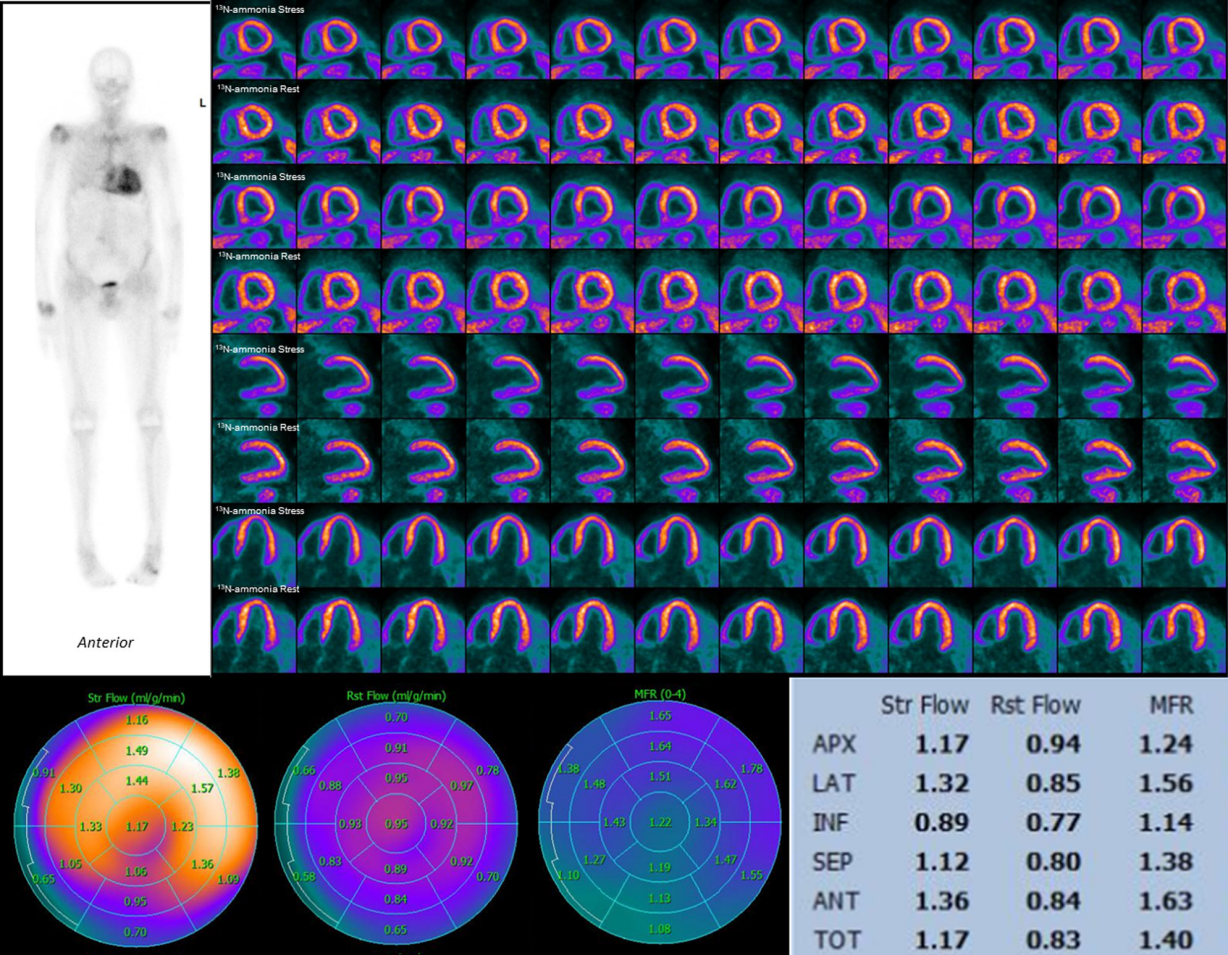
ATTR Amyloidosis and CMD. A 82-year-old patient with left ventricular hypertrophy on TTE was assessed for presence of cardiac amyloidosis. Upper left panel depicts planar whole-body scintigraphy with a bone tracer (Tc-99m-DPD) revealing increased myocardial tracer uptake consistent with cardiac ATTR amyloidosis. Upper right panel shows ^13^N-ammonia PET perfusion images (short axis, horizontal and vertical long axis slices) demonstrating no regional perfusion defect in stress or rest. Quantitative PET analysis shows a significant reduction of hyperemic MBF and a severely reduced global MFR (lower panels)
